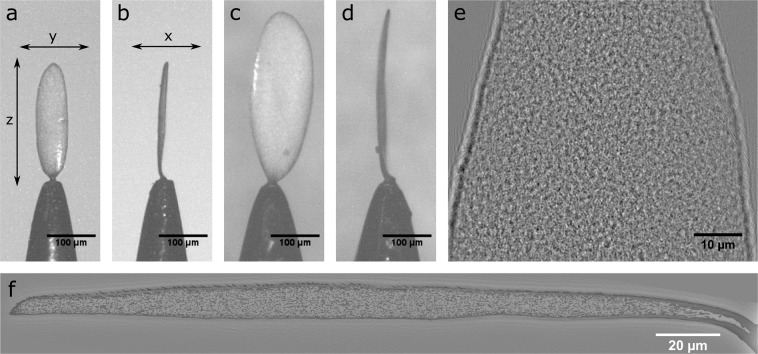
Various optical, x-ray and reconstructed images of the beetle scales. (a,b) The mounted Cyphochilus scale *including a* coordinate system *for reference*. (c,d) A mounted L. stigma scale. (e) An X-ray projection image of scan 2 of the Cyphochilus scale. (f) A single slice through the xz plane of the assembled and aligned Cyphochilus matrix.
